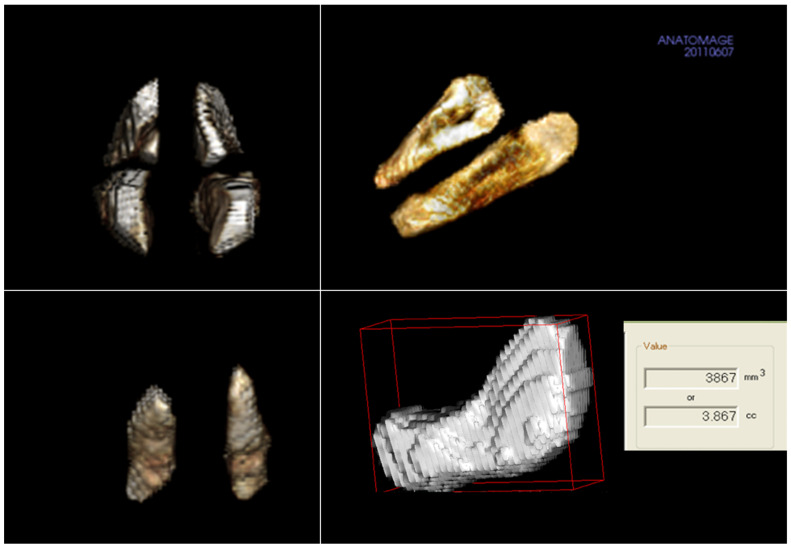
Three-dimensional volume renderings and segmentation of the INC, and MNC obtained from CBCT images. The segmented structures represent the measured volumes of the INC, as part of the volumetric assessment process.
